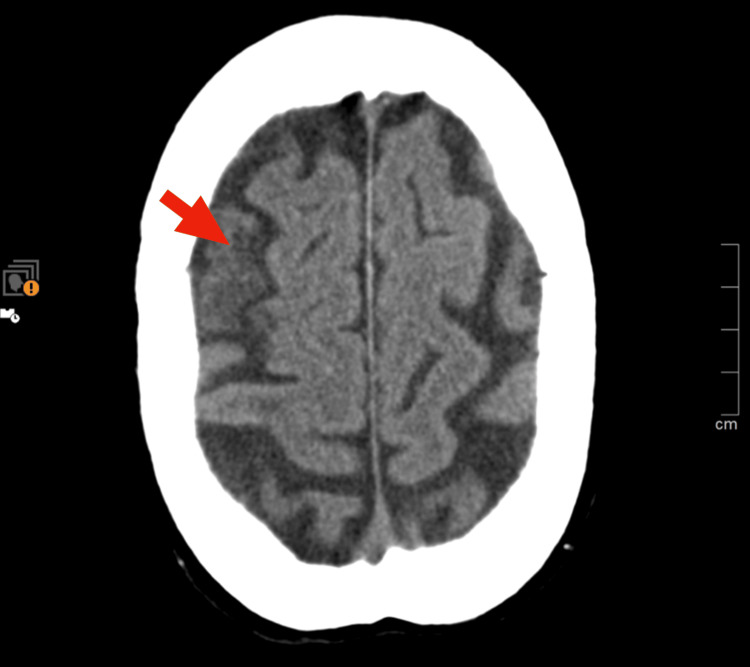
Cranial (CT) scan shows chronic ischemic changes in the right frontocortical and subcortical regions
